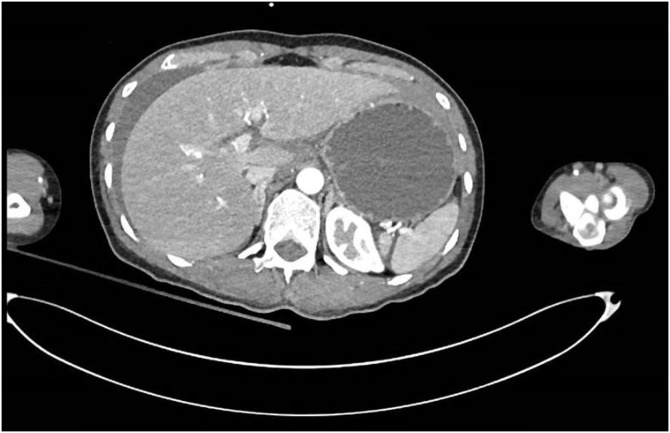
Axial CT showing free fluid around the liver.
